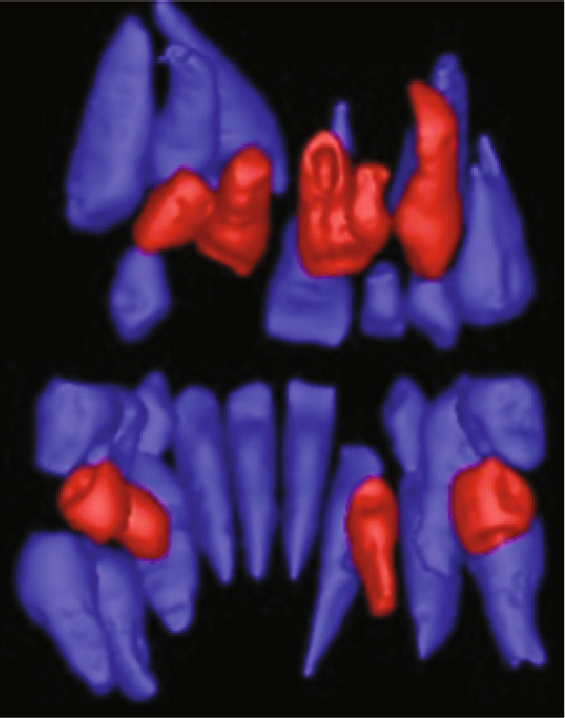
A segmented picture of the preoperative cone-beam computed tomography (CBCT) showing the positions of the nine supernumerary teeth (colored red) from a posterior view.
